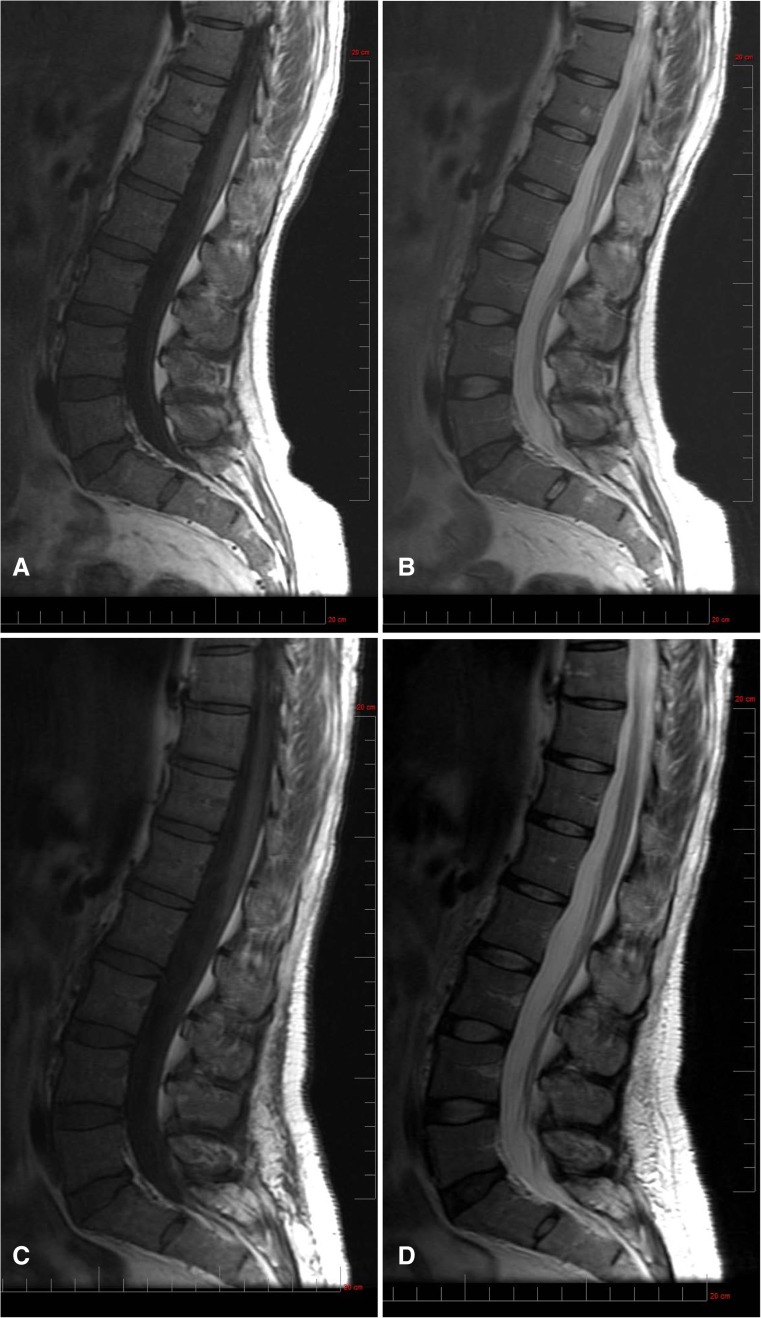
Assessment of olipudase alfa on bone marrow burden. Femur. Bone marrow burden changes in the coronal femur of patient 2, (female, 32 years old at baseline). Note the extent of hypointensity of the proximal epiphysis bone marrow at screening in the T1-weighted (a) and T2-weighted (b) images compared with the reduced amount and slightly hypointense diaphyseal bone marrow following 30 months of treatment (T1-weighted, c and T2-weighted, d). Full vertical scale bar, 20 cm. Spine. Bone marrow burden in the sagittal lumbar spine of patient 2. At screening, diffuse infiltration of the bone marrow is observed with T1-weighted isointensity of the non-diseased intervertebral discs (a) and T2-weighted hyperintense signal intensity of presacral fat (b). After 30 months of treatment, the infiltration of the bone marrow remains unchanged (T1-weighted, c) while the presacral fat is improved to slightly hyperintense (T2-weighted, d). Full vertical scale bar, 20 cm
